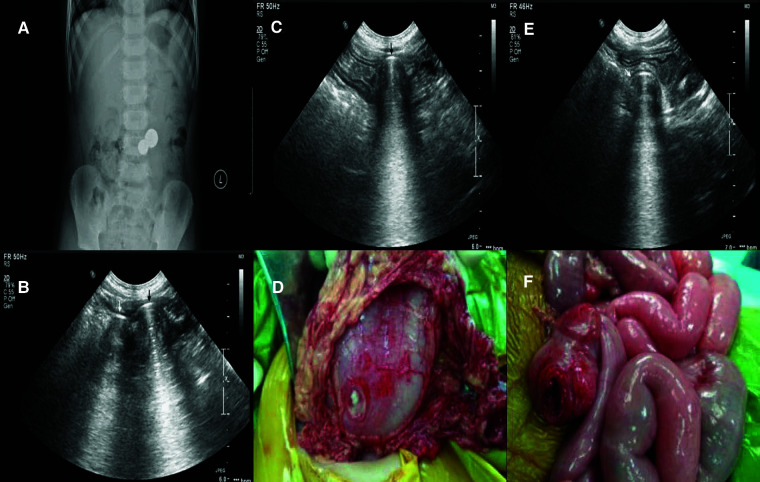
Multiple magnets in the gastrointestinal of a 6-year-old boy. The plain film indicated two magnets attracted together (A), as indicated by the sonogram. (B) The sonogram revealed one magnet (black arrow) in the stomach (C) and the other magnet (white arrow) in the colon. (E) This indicates wall entrapment, which was confirmed by surgery. Perforation of the stomach (D) and colon (F) were found during surgery.
